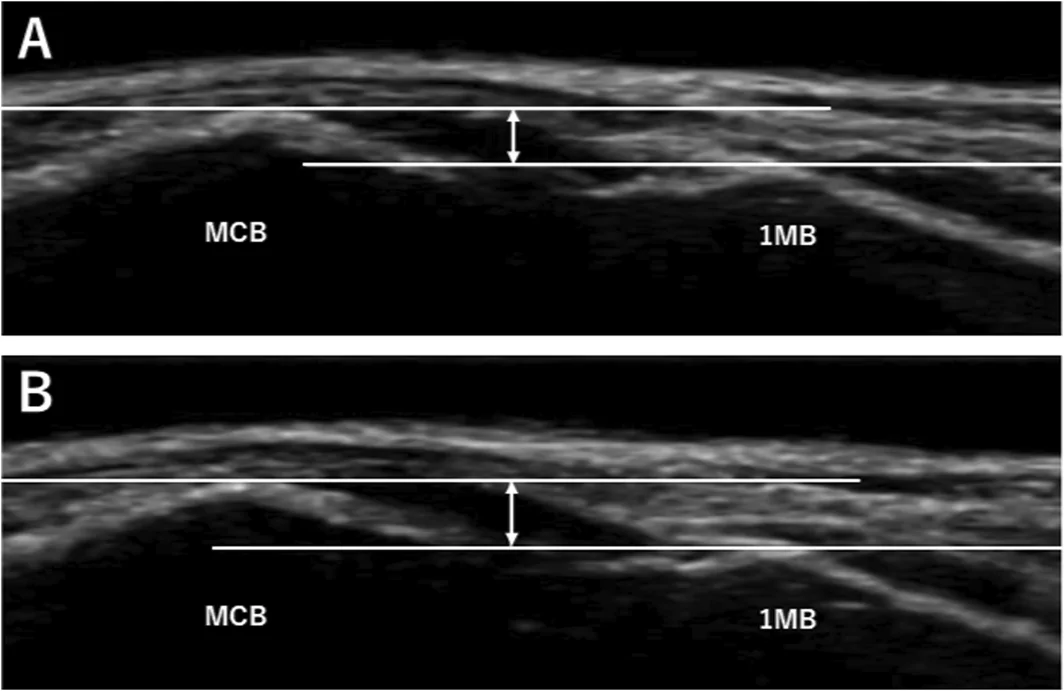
Long‐axis ultrasound images of the dorsal aspect during TMT joint displacement assessment. The white line indicates the horizontal reference line aligned with the most prominent points of the medial cuneiform and first metatarsal. The arrow indicates the vertical distance between the medial cuneiform and the first metatarsal. (A) Before and (B) after stress loading. 1 MB, first metatarsal bone; MCB, medial cuneiform bone.
